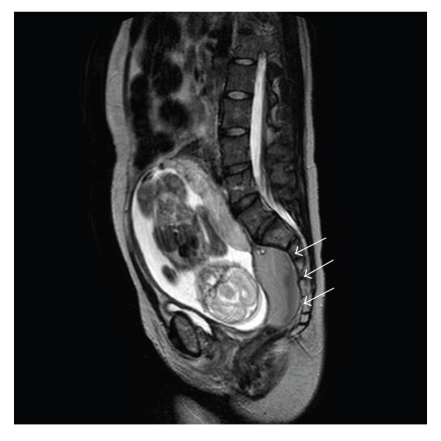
MRI at 21 weeks of gestation. Arrows show location of intra-abdominal bleeding.
